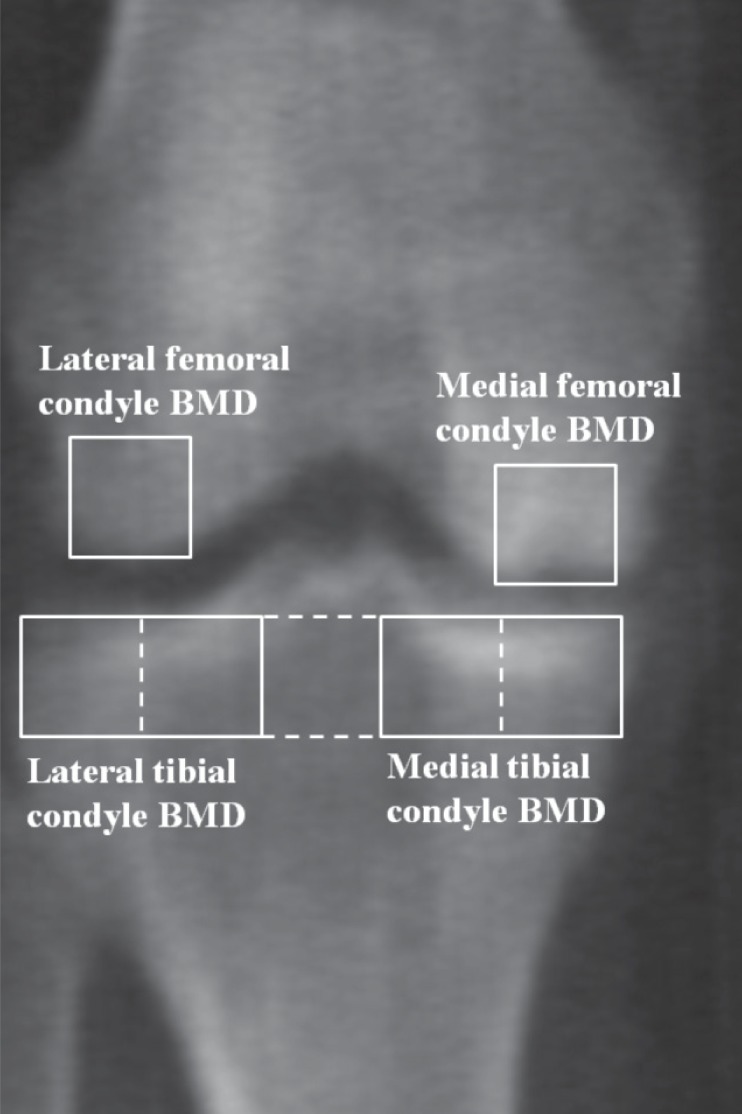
An AP dual X-ray absorptiometry image of the right knee of a 74-year-old woman 7 weeks after the onset of pain (the same patient as in Figure 2) showing a necrotic lesion surrounded by a sclerotic area in the medial femoral condyle. In the tibial condyles, five square regions of interest were marked on the frontal view. A line extending to the lateral and medial edges of the proximal tibia was divided into 5 equal lengths and 5 square regions of interest were marked underneath it. The medial tibial condyle BMDs in the 2 medial square regions of interest and the lateral tibial condyle BMDs in the 2 lateral square regions of interest were calculated for the tibia. In addition, the lateral and medial femoral condyle BMDs were calculated in square regions of interest of the same size as those on the tibial condyles located on a line passing through the tips of the medial and lateral condyles, with the midpoints of their distal sides at the points of contact.
